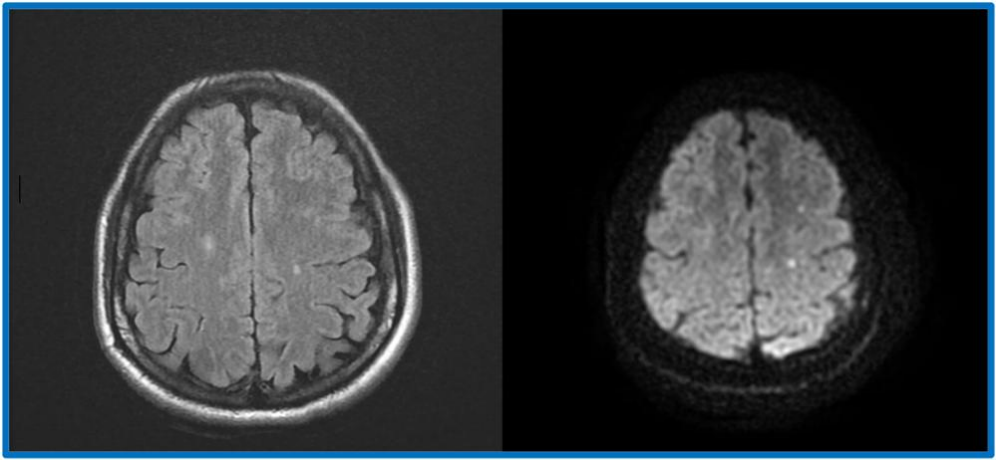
Patient’s cerebral MRI showing multiple small acute infarcts at the bi-hemispheric anterior junction.
